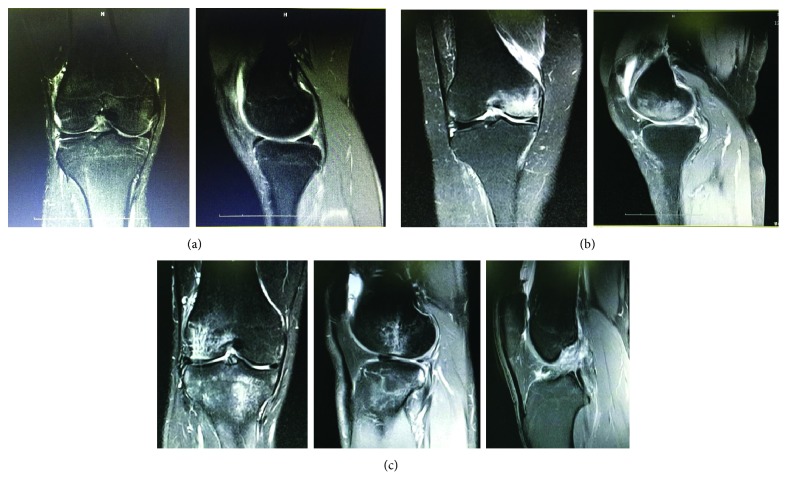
(a) Case 1: male, 21 years old, long distance runner, right knee pain, and swelling after knee longitudinal impact injury in one long-distance race. MRI showed that medial femur and medial tibia had mild bone bruise, bone marrow edema, and mild joint effusion. (b) Case 2: male, 77 years old, right knee pain, and swelling after one falling longitudinal impact injury at one meter high. MRI showed widely that medial femoral condyle had bone marrow injury and edema. Medial tibia had mild bone bruise and joint effusion. (c) Case 3: male, 22 years old, soldier, right knee pain, swelling, and movement dysfunction after knee longitudinal impact injury in one military training. MRI showed widely that medial tibia had bone marrow edema, meniscus injury, and rupture of anterior cruciate ligament.
